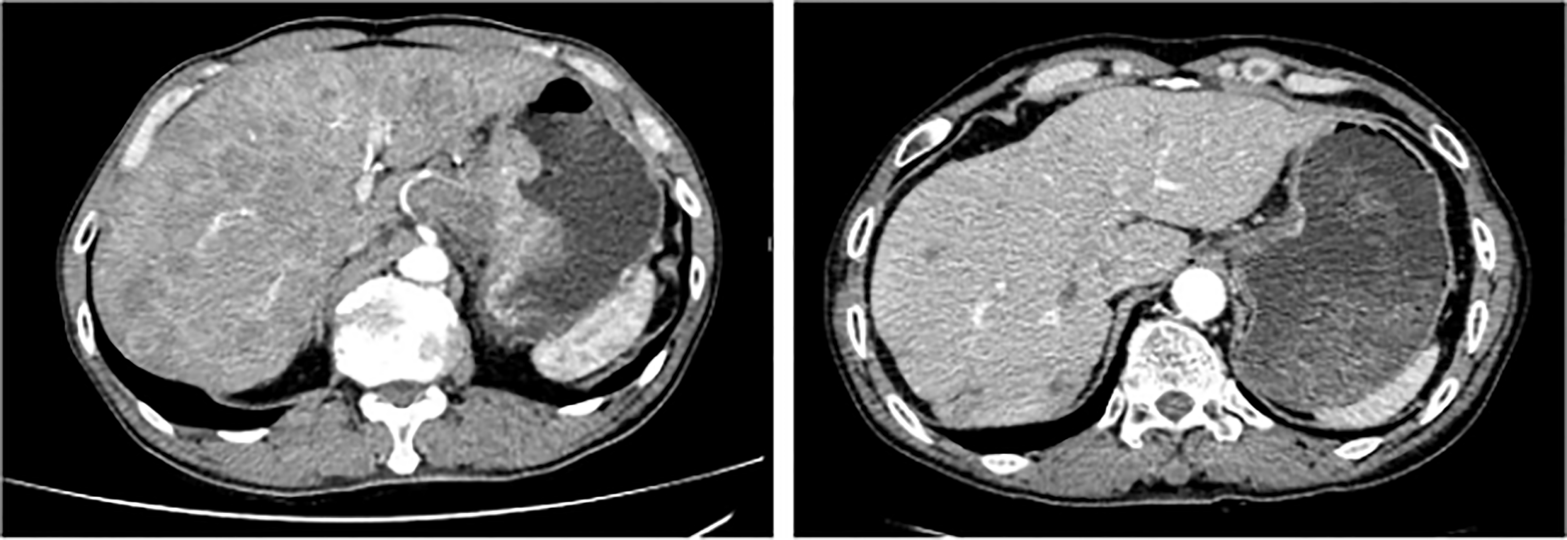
Computed tomography (CT) scan in a HER2-positive patient with high TMB achieving CR to HXP. The image on the left was at the time of treatment start, and the image on the right was at the time of CR achievement. HER2, human epidermal growth factor receptor 2; TMB, tumor mutational burden; CR, complete response; HXP, trastuzumab, capecitabine, and cisplatin.
